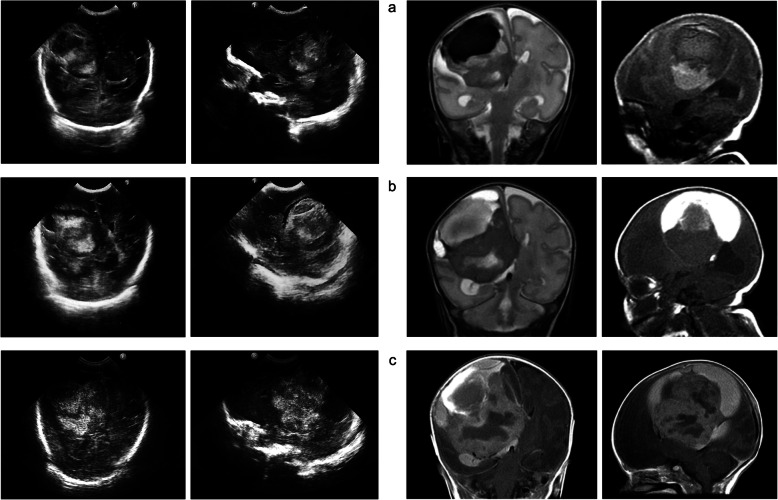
Cranial ultrasound (left columns) and magnetic resonance imaging (right columns) on day 1 (a), 18 (b) and 28 (c)
